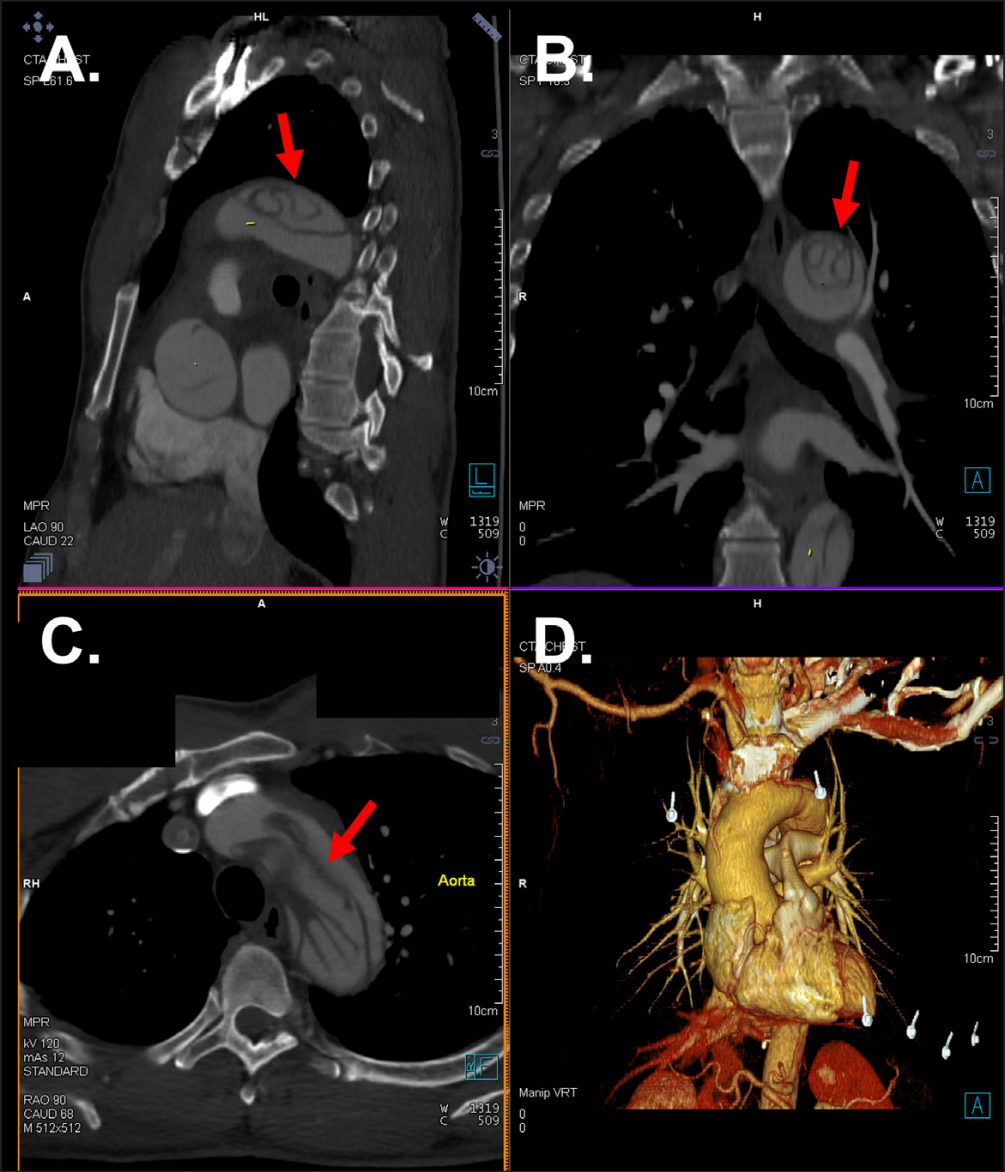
Aortic arch dissection. Sagittal (A), coronal (B), and transverse (C) computed tomography showing complex dissection flap at the level of the aortic arch (arrow). Three-dimensional reconstructed computed tomography is provided (D).
